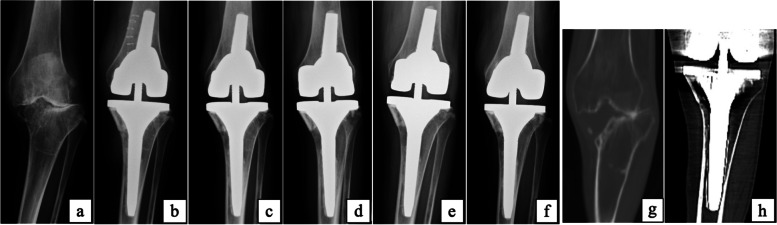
Pre- and postoperative follow-up radiographs and computed tomography (CT) scan (a) Preoperatively. (b) Immediately after surgery. (c) 6 months after surgery. (d) 3 years after surgery. (e) 7 years after surgery. (f) Final follow-up (11.5 years). (g) CT scan preoperatively. (h) CT scan at 3 years after surgery
